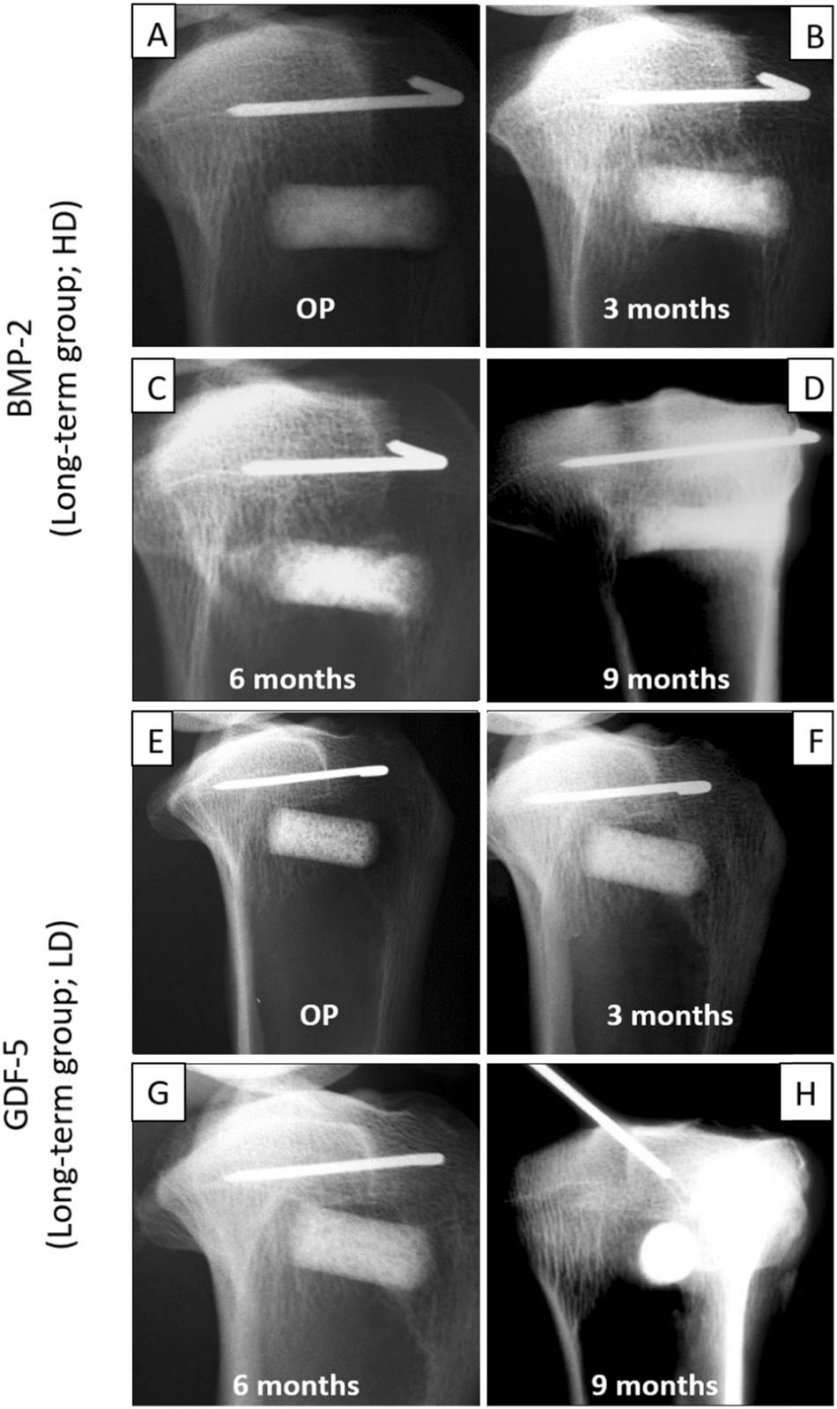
Time course of the effects of the BMP-coated implant cylinders in the tibial head concerning the “best-matching dosages of BMP-2” (high-dose [HD]; 250 µg (A–D)) and “GDF-5” (low-dose [LD]; 125 µg (E–H)). X-rays were taken immediately after surgical insertion (OP) and at subsequent 3 month intervals
